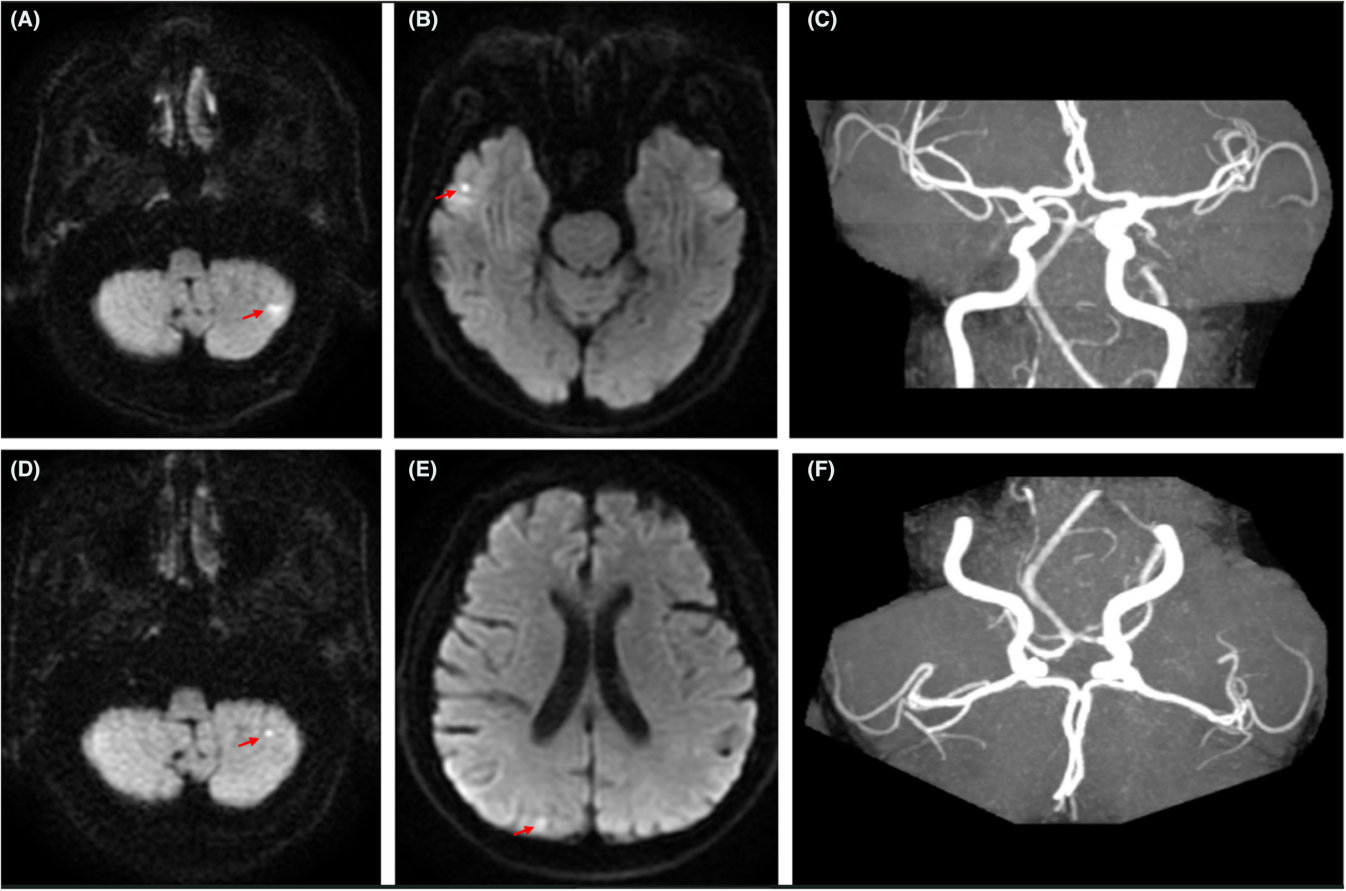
Brain MRI. (A, B) DWI of the brain on day 3 showed patchy high signal intensities in the right temporal lobe and left cerebellum. (C, F) No obvious blood vessel obstruction or stenosis was observed by magnetic resonance angiography. (D, E) DWI of the brain on day 34 revealed slightly hyperintense signals in the left cerebellum and right occipital lobe. (magnetic resonance imaging, MRI; Diffusion‐weighted imaging, DWI)
